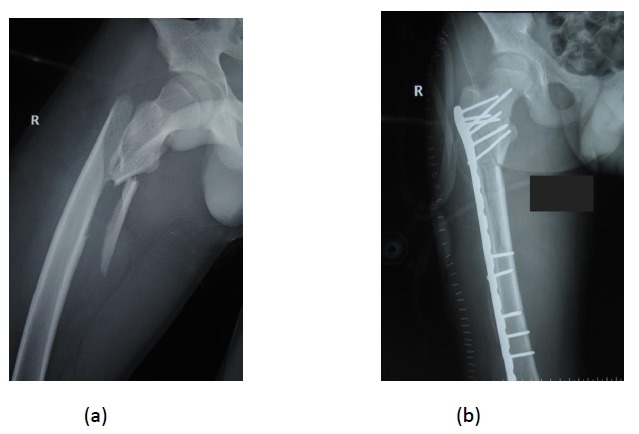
Preoperative and post operative radiographs of a comminuted subtrochanteric fracture with extension into the femoral shaft managed by submuscular bridge plating.
